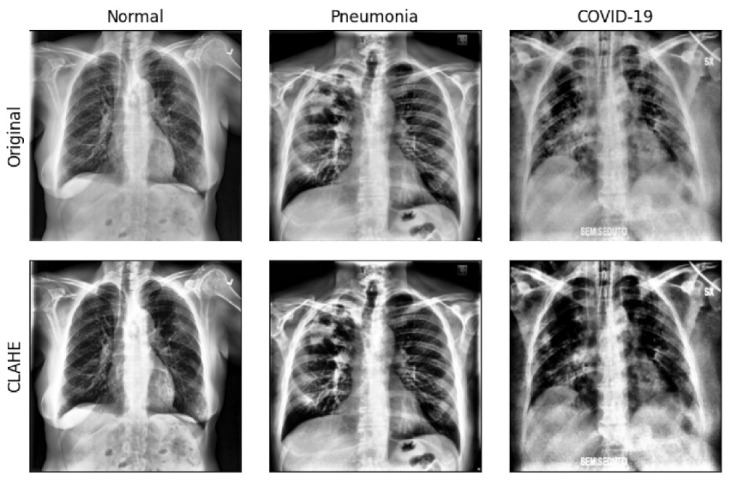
Sample images after applying contrast correction by contrast limited adaptive histogram equalization (CLAHE) and the semantic segmentation of lung on original chest X-ray (CXR) images.
